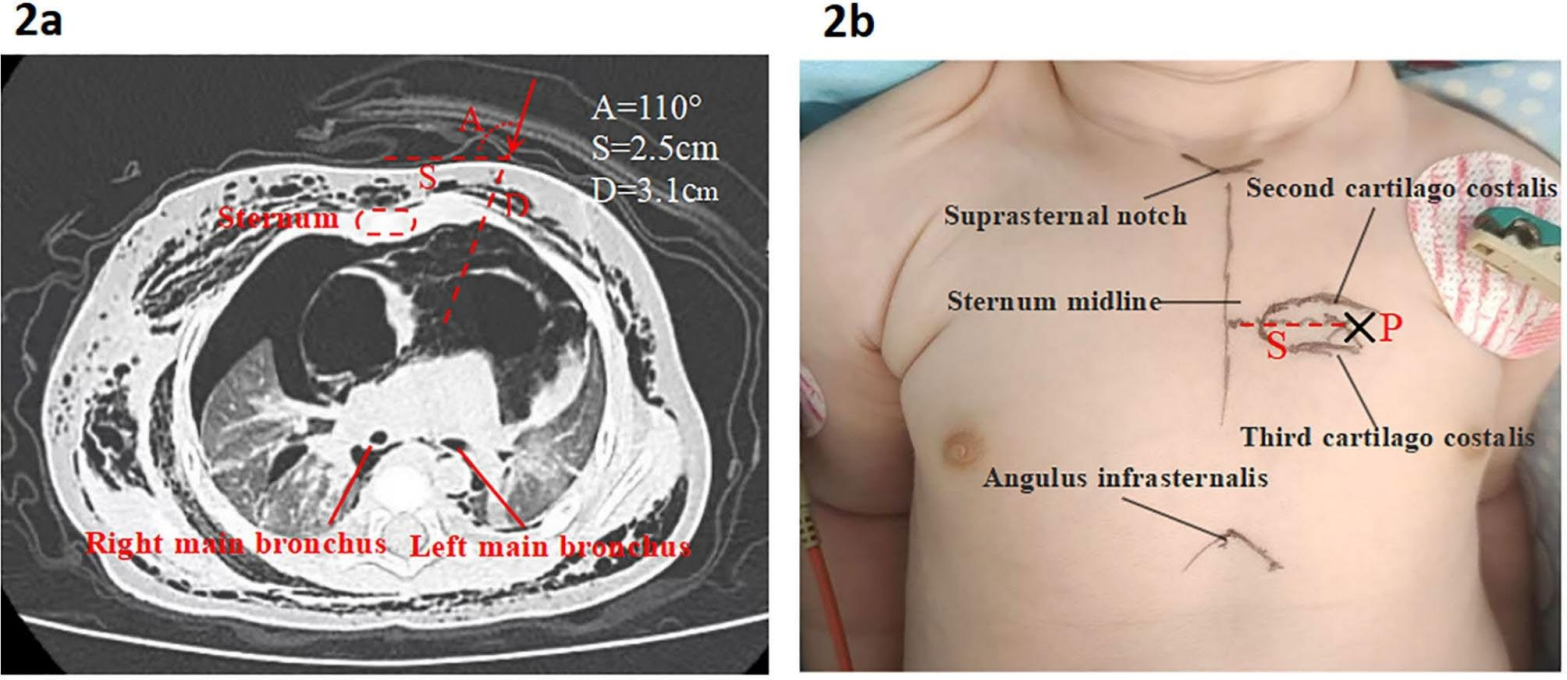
2a: Plan the puncture path using preoperative chest computed tomography. Extensive mediastinal tension emphysema compressing the mediastinal vessels and both lungs, the trachea is pushed posteriorly. Extensive lateral subcutaneous emphysema. 2b: Skin surface markers of the puncture site. A: The angle of needle insertion; D: The depth of pneumomediastinum; S: The spacing between the puncture site and the sternum midline. P: The puncture site
